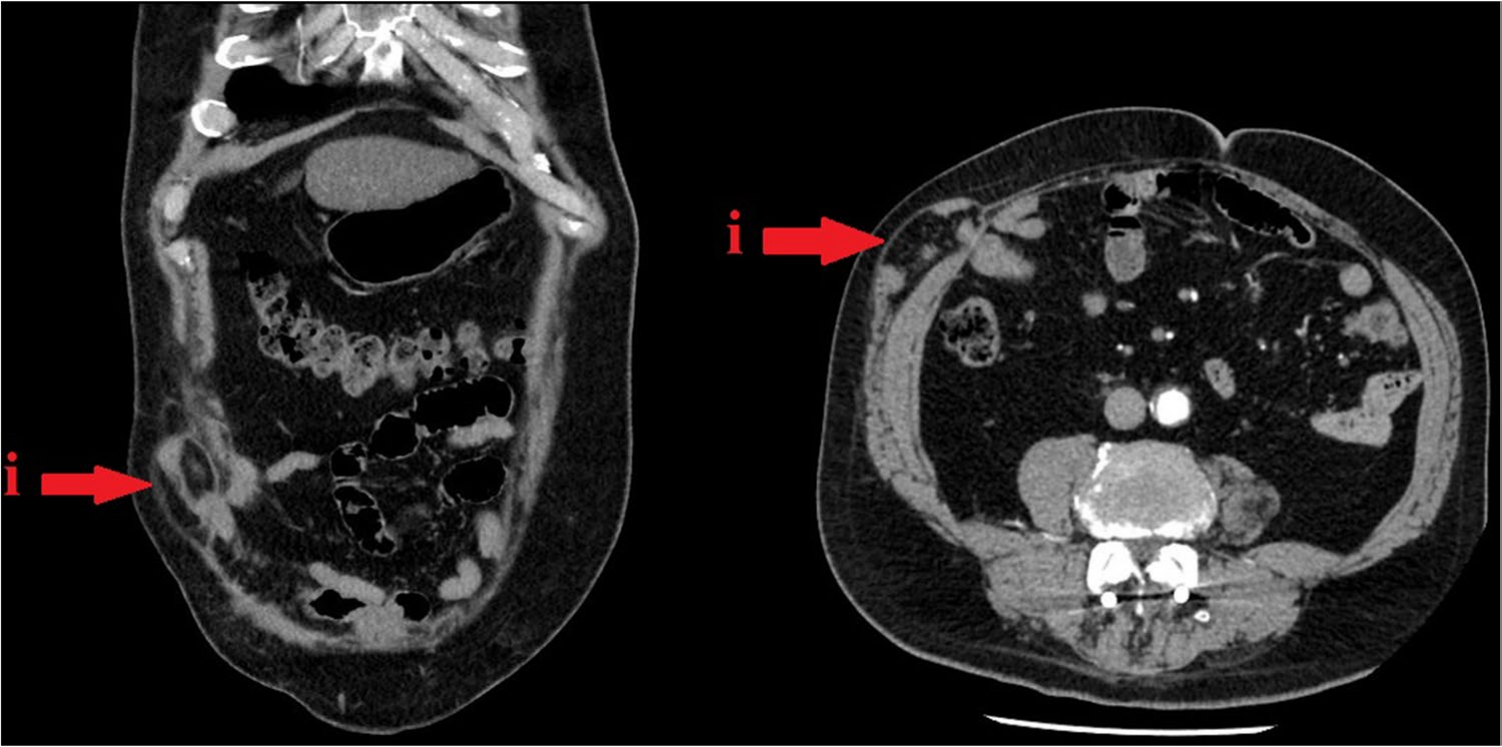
Massive incisional hernia (i) through abdominal wall on CT scan (coronary and axial projection) of patient with personal history of previous abdominal surgery - open cholecystectomy (picture from author‘s personal collection)
